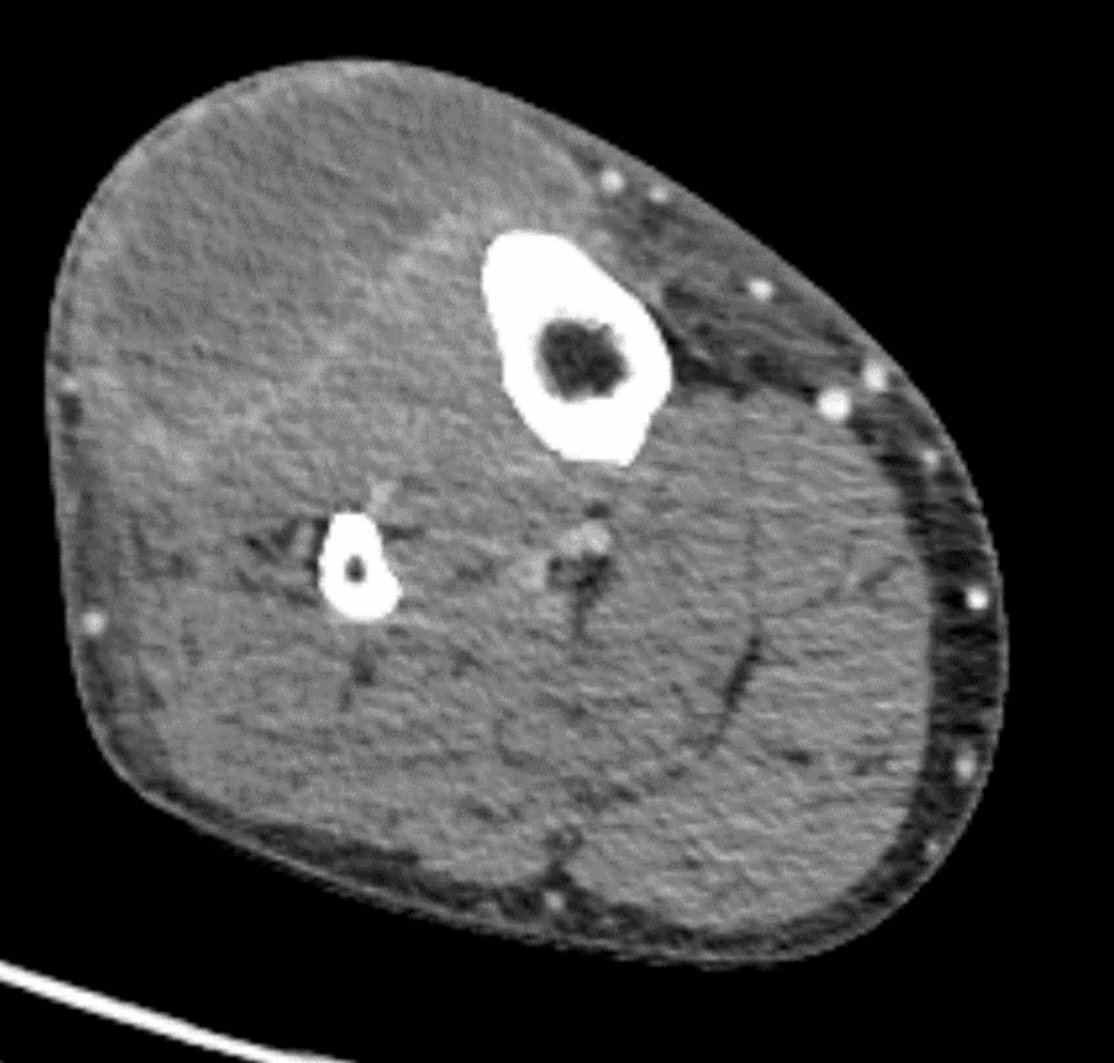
CT angiography of the right lower leg Heterogeneous-appearing mass within the anterior right lower leg has increased in size from November 21, 2023
